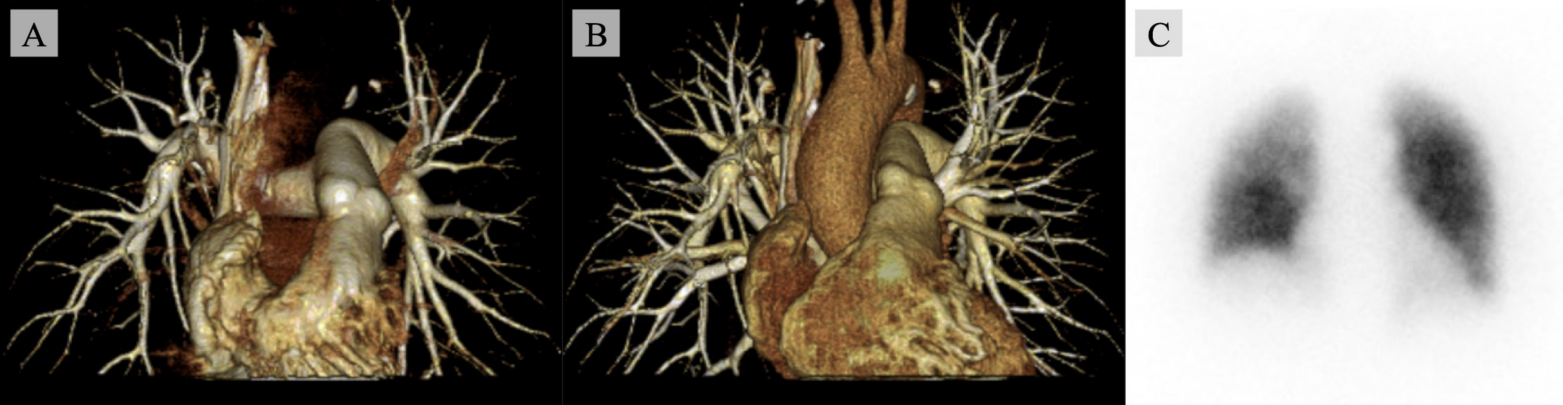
(A) Four‐dimensional reconstructed enhanced chest computed tomography (4D‐CT) at 12 months after surgery shows no abnormal vessels in the pulmonary arterial phase. (B) In the pulmonary venous phase of 4D‐CT, no abnormal vessels are detected. (C) Pulmonary blood flow scintigraphy at 12 months after surgery shows no perfusion defects.
